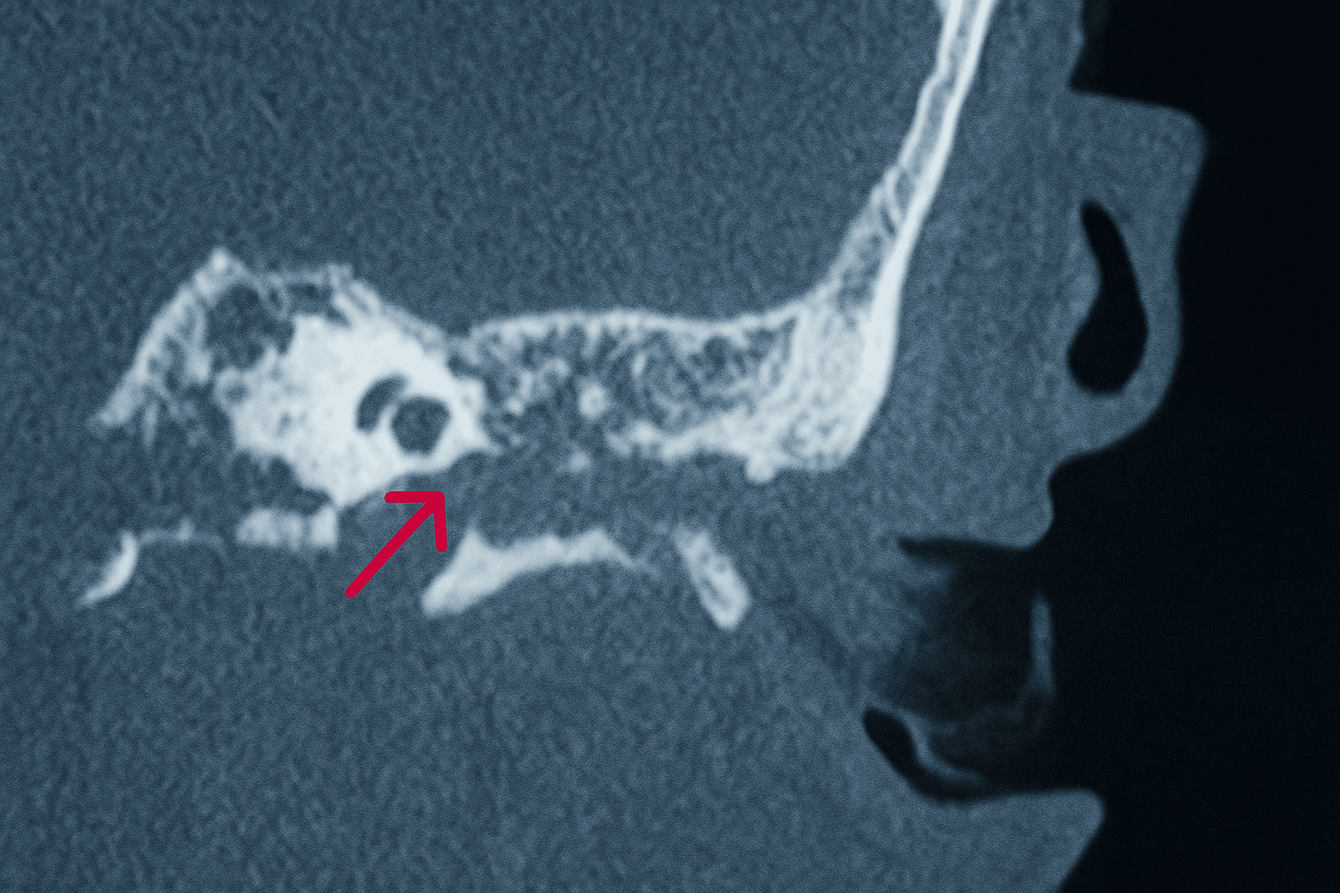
Coronal CT image in the bone window demonstrating lysis in the inferior wall of the left tympanic cavity (red arrow).
